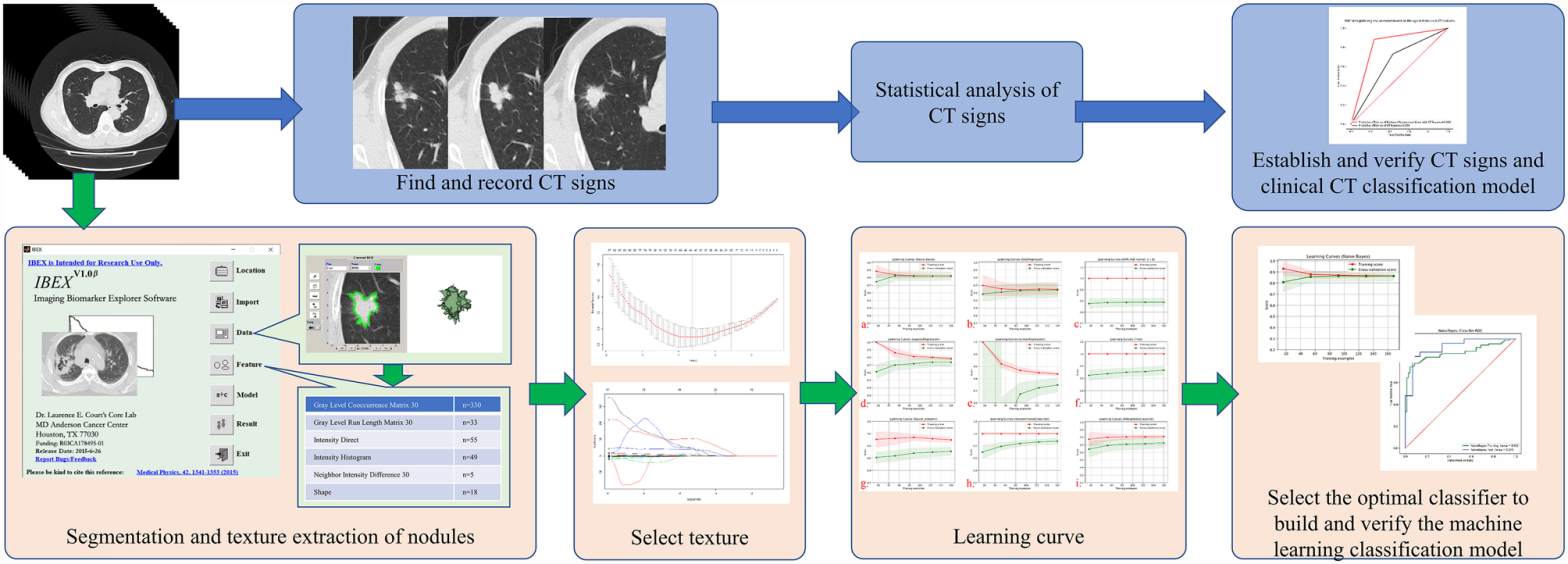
Research path map. Blue path, routine clinical CT diagnosis process. The features were found from CT images, and the differential diagnosis models were established to evaluate CT features and clinical CT diagnosis. Green path, machine learning process. The texture features are extracted from CT images by IBEX software. The extracted texture features are reduced by LASSO regression. The best classifier is selected by learning curves of machine learning, and the machine learning classification model is established and tested.
